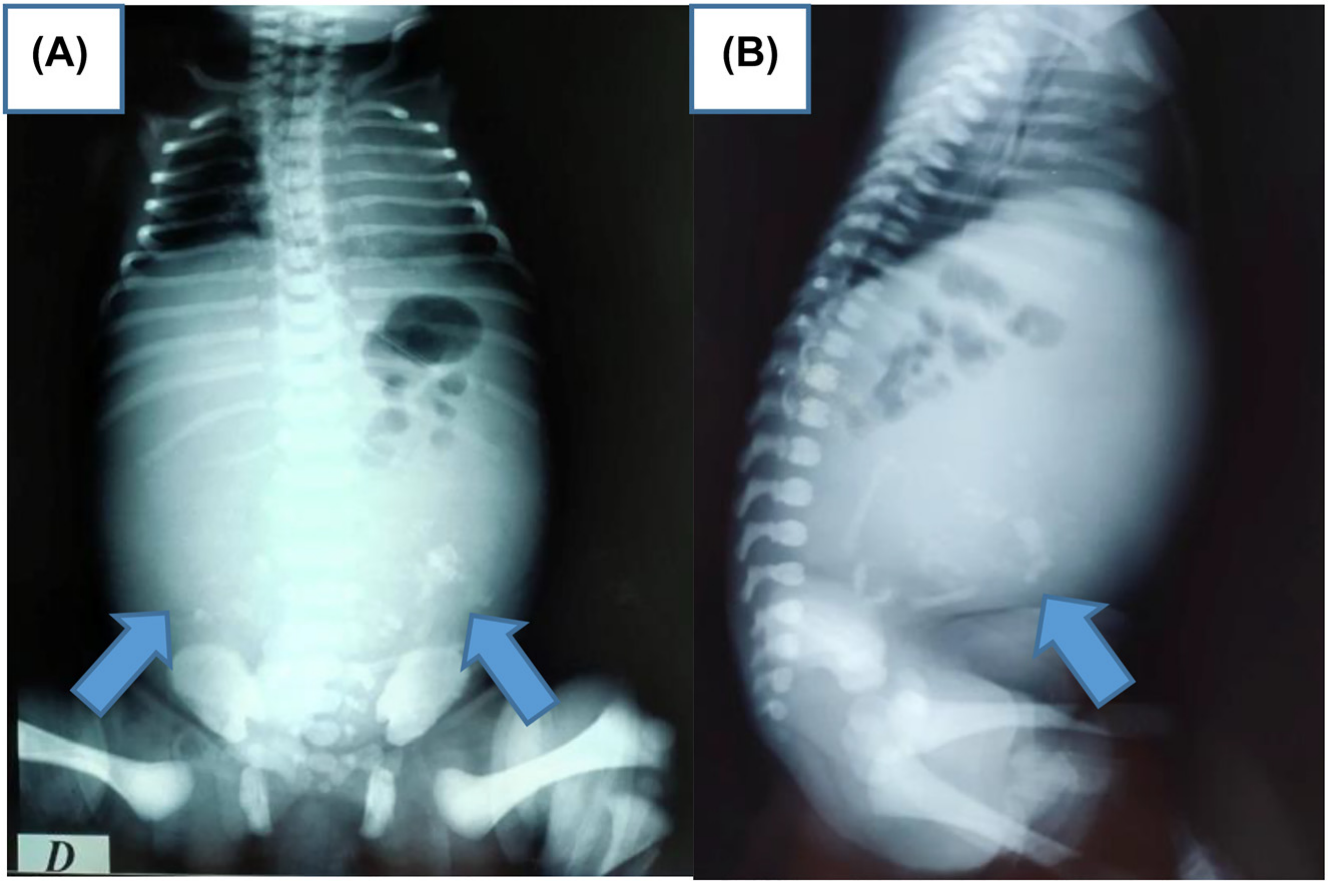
(A, B): Radiograph showing fetus skeleton inside the abdomen of the newborn resembling well-formed limb bones and rib (arrows).
